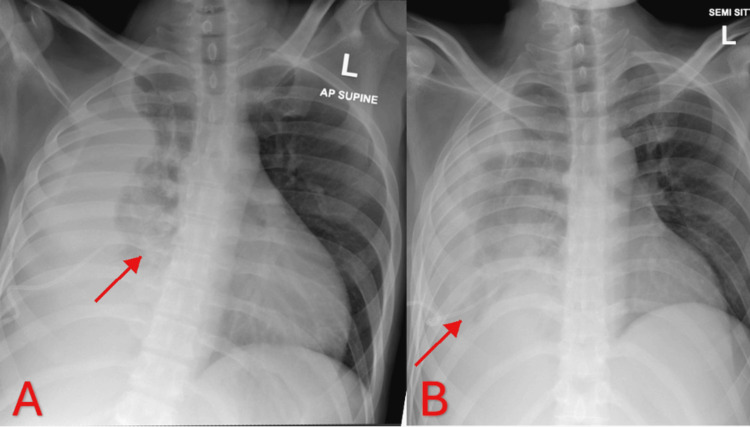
Serial chest radiographs demonstrating the clinical course (A) Anteroposterior (AP) supine chest radiograph on admission demonstrating complete opacification of the right hemithorax with contralateral mediastinal shift, consistent with a large right-sided pleural effusion. (B) Semi-sitting anteroposterior chest radiograph following chest tube insertion, showing partial re-expansion of the right lung with residual right lower zone opacity and a more central mediastinal position, indicating interval improvement.
